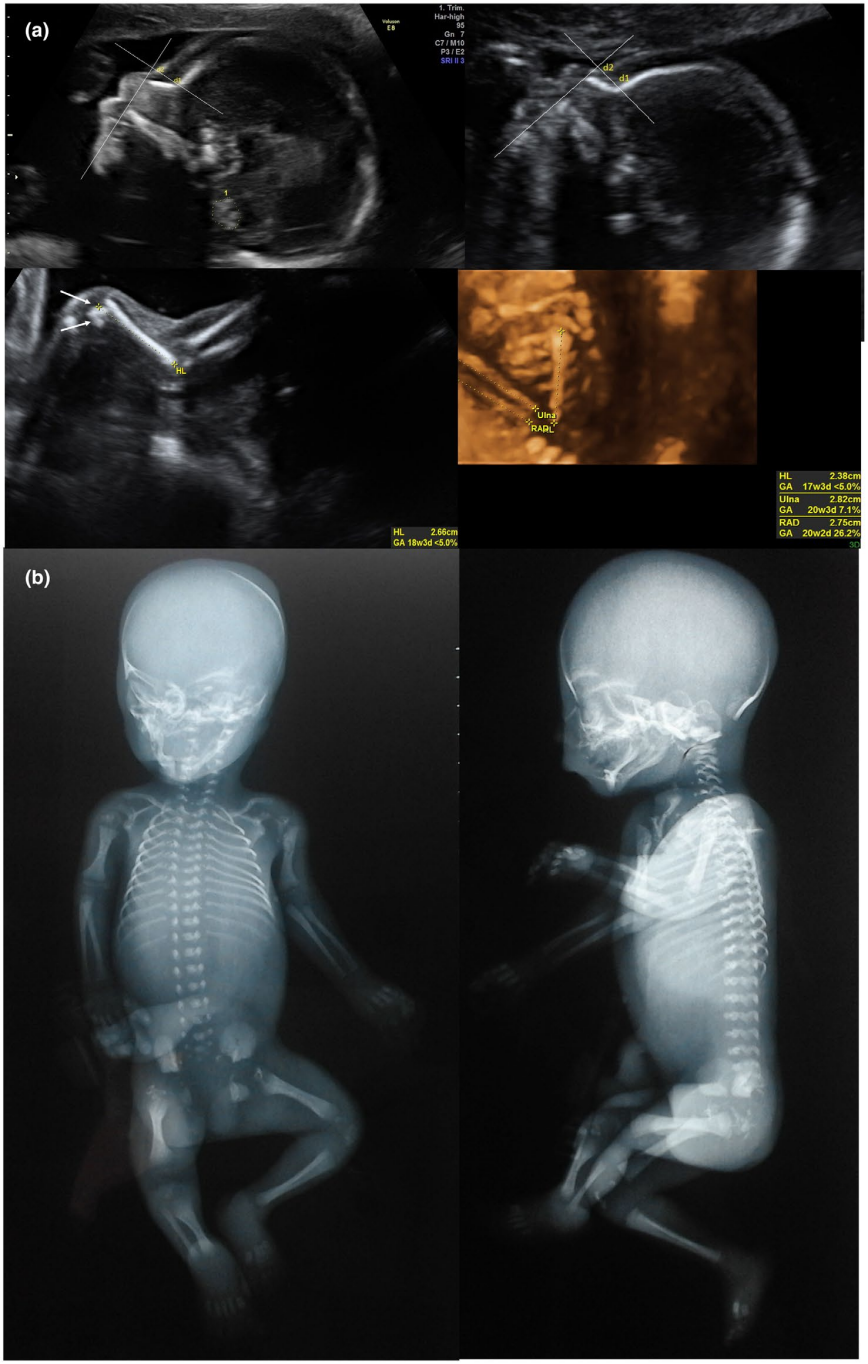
Sagittal ultrasound image of a foetus at 21 weeks 4 days of gestational age (a) and 17 weeks 5 days (a.1) showing prefrontal oedema (d1‐d2) with normal nasal bone and normal frontonasal angle, an important ultrasound sign for the differential diagnosis of the RCDP sub‐type. Longitudinal 2D (a.2) and 3D (a.3) ultrasound image of the upper long bones at 21 weeks 4 days of gestational age showing the rhizomelia and the epiphyseal stippling (arrows). Radio and ulna biometry is in accordance with the gestational age. (a.2;a.3), (b;b.1) Postnatal radiographs confirming the shortness of both humeri and the presence of calcified punctures in humeral periarticular cartilage. RCDP, Rhizomelic chondrodysplasia punctata
